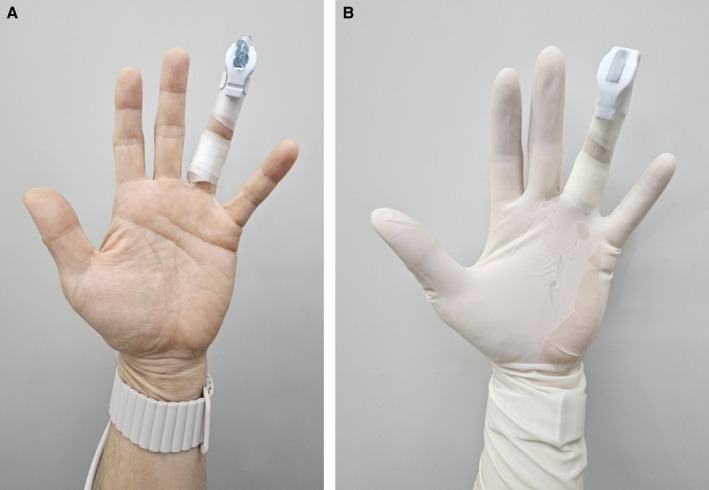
Method of wearing the ultrasound probe.
